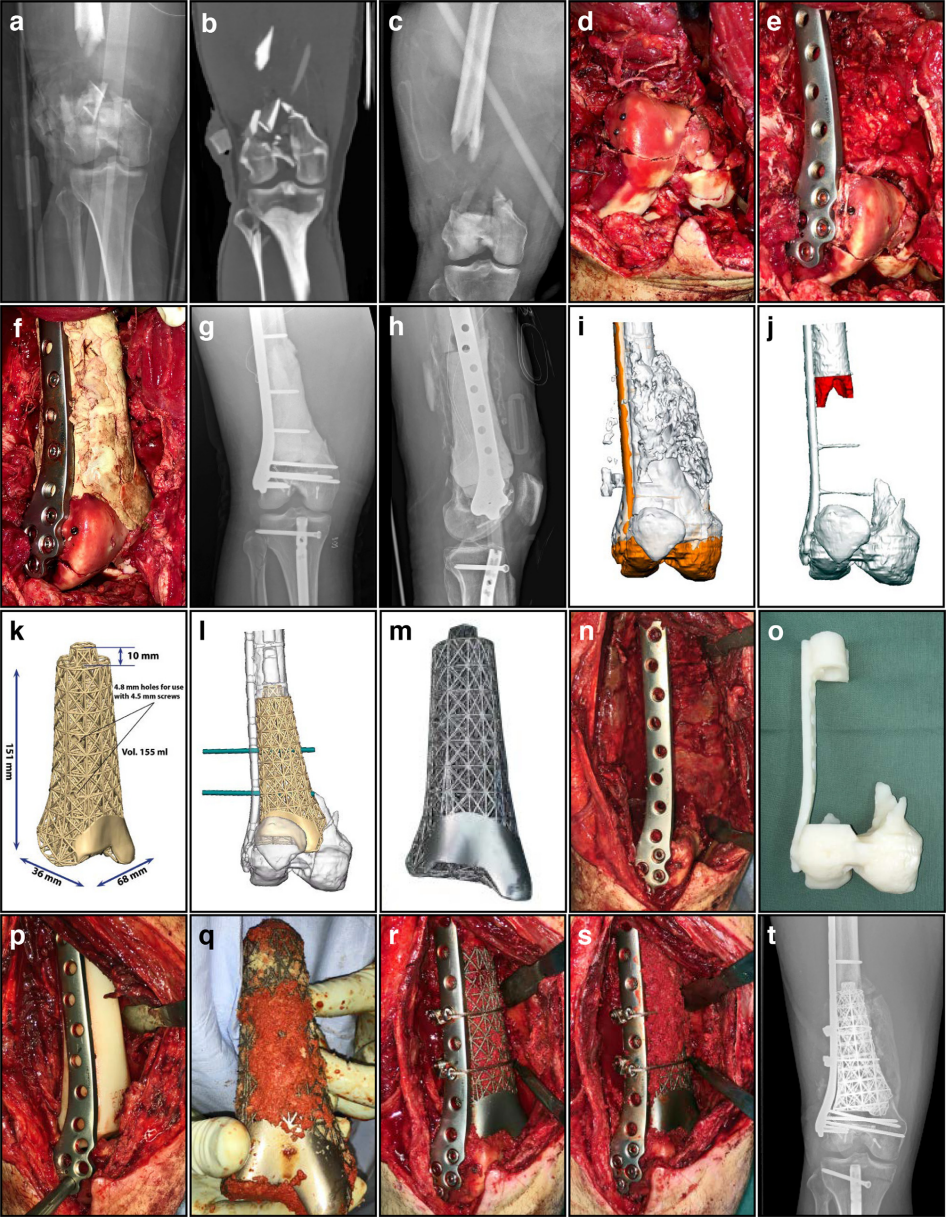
Metadiaphyseal distal femoral segmental defect (15.1 cm) – Grade 3A open fracture. (a) AP radiograph of this Grade 3A open R distal femur fracture immediately following the injury. (b) Initial CT scan demonstrating loss of bone stock and cartilage centrally, with less than 6 mm of bone adjacent to the intercondylar notch. (c) AP radiograph of the distal femur after initial debridement and spanning external fixation. (d) Intra-operative photos during the 1st stage, with reconstruction of the highly comminuted intra-articular extension of the fracture. (e) Intra-operative image showing the fracture reduced anatomically and then stabilized with a locked plate. (f) Intra-operative image during the 1st stage showing the PMMA spacer temporarily filling the defect, augmenting mechanical support of the distal segment while also providing a surface for the development of an induced membrane. (g) AP radiograph following stabilization with a lateral locked plate, incorporating an antibiotic-loaded PMMA spacer. (h) Lateral radiograph of the distal femur with plate and temporary PMMA spacer. (i) The patient-specific 3D printed titanium truss implant was produced after first conducting a virtual surgical procedure. (j) Additional bone was resected (red) to facilitate the actual procedure and to maximize contact and inherent stability. (k) Dimensions of the 3D printed titanium truss implant, designed with a tapered intramedullary extension proximally that increases stability significantly. (l) The truss implant design included trajectories for screw holes that correspond to the existing implant (green). (m) The final implant was produced with a polished surface to articulate with the patella, indicating extent of cartilage loss. The design of the implant very closely mimics the contours and dimensions of the original bone. (n) Intra-operative image during the second stage, with carefully opened and preserved membrane after the PMMA spacer was removed. (o) 3D printed acrylic model of the anticipated final skeletal defect, used to confirm satisfactory fit and alignment of the implant. (p) Intra-operative image showing insertion of a plastic template to assess the adequacy of the final resection, used as a trial before inserting the implant. (q) Before implantation, the truss construct was filled with autologous and allogeneic cancellous bone graft mixed with powdered vancomycin, then manually packed into the open cells of the truss implant. (r) Intra-operative image showing the truss implant filled with bone graft and inserted into the defect, with additional fixation including two cerclage cables. (s) Intra-operative image during the second stage, showing additional bone graft packed over the anterior and medial aspects of the truss implant after definitive fixation was completed. (t) AP radiograph demonstrating early incorporation of the bone graft, with a solid column of dense bridging bone visible medially four months after the procedure.
